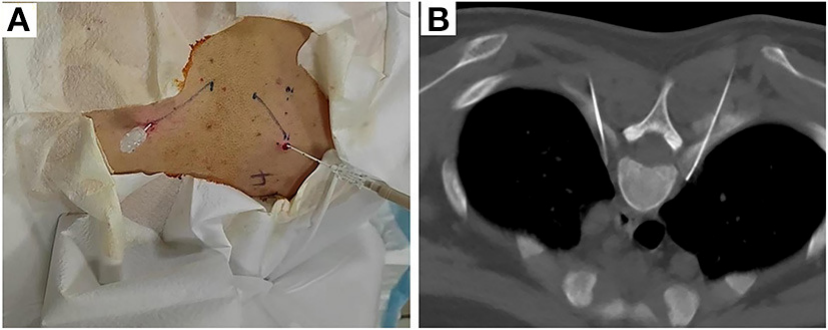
Body surface localization (A) and radiofrequency targeting points (B) of CT-guided thoracic sympathetic nerve radiofrequency thermocoagulation using the T4 segment as an example.
